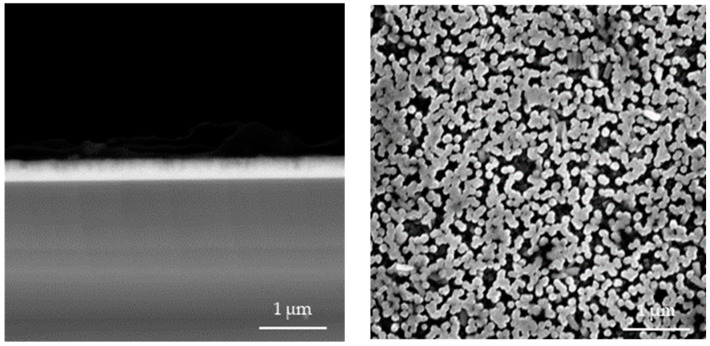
SEM images of nanorods obtained with 4 min of ultrasound and dried for 1.5 h (left: cross-section image; right: surface image).
